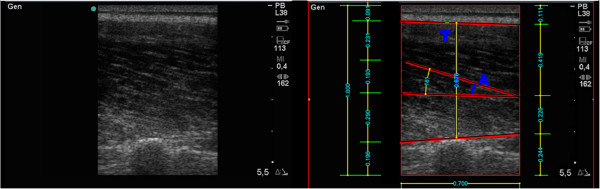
Example architecture variables measure from ultrasound image. A: Pennation Angle. T: Muscular Thickness.
